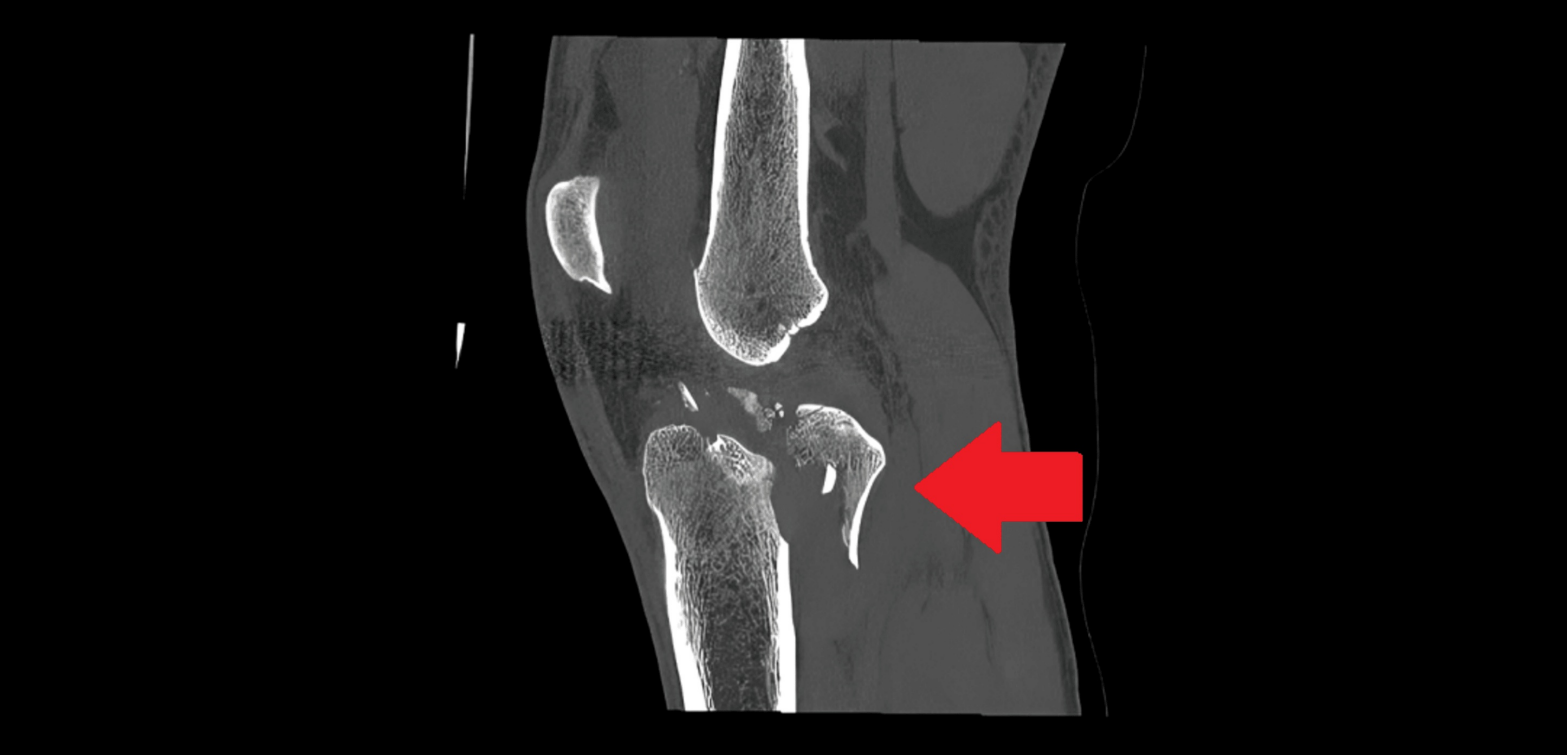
A CT scan showing the fracture, with sagittal view of the left knee
